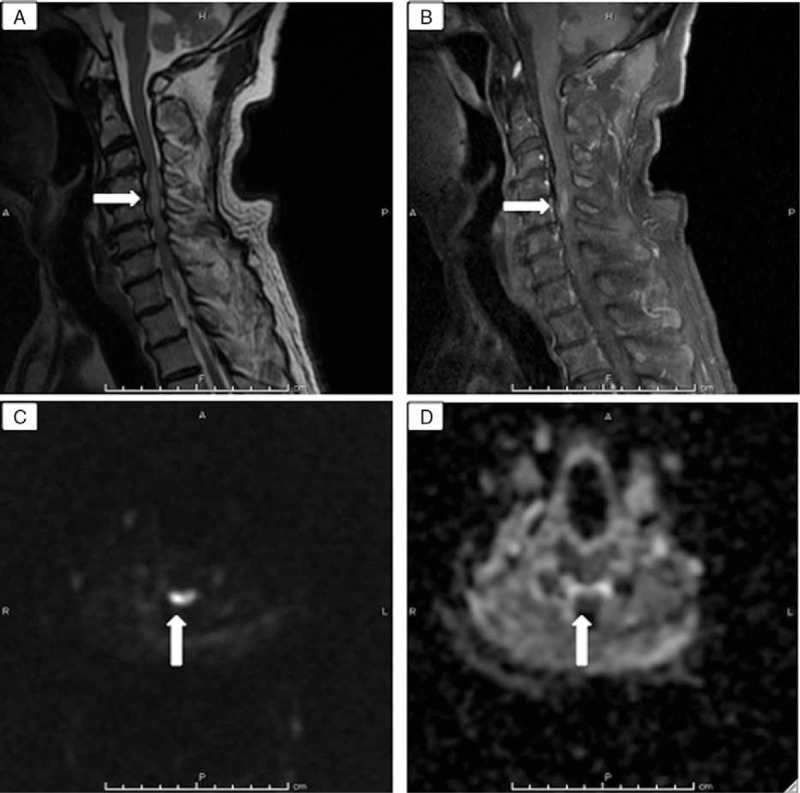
Magnetic resonance imaging (MRI) findings on hospital day 8. High signal intensity at C4/5 (arrows) on (A) sagittal contrast-enhanced T2-weighted (3000/99.21 [TR/TE]) image, (B) T1-weighted (516.67/11.96 [TR/TE]) image, and (C) axial diffusion weighted imaging (DWI; 4775/68.30 [TR/TE]) image. D, Axial ADC (4775/68.30 [TR/TE]) map shows a dark area at C4/5 (arrow).
